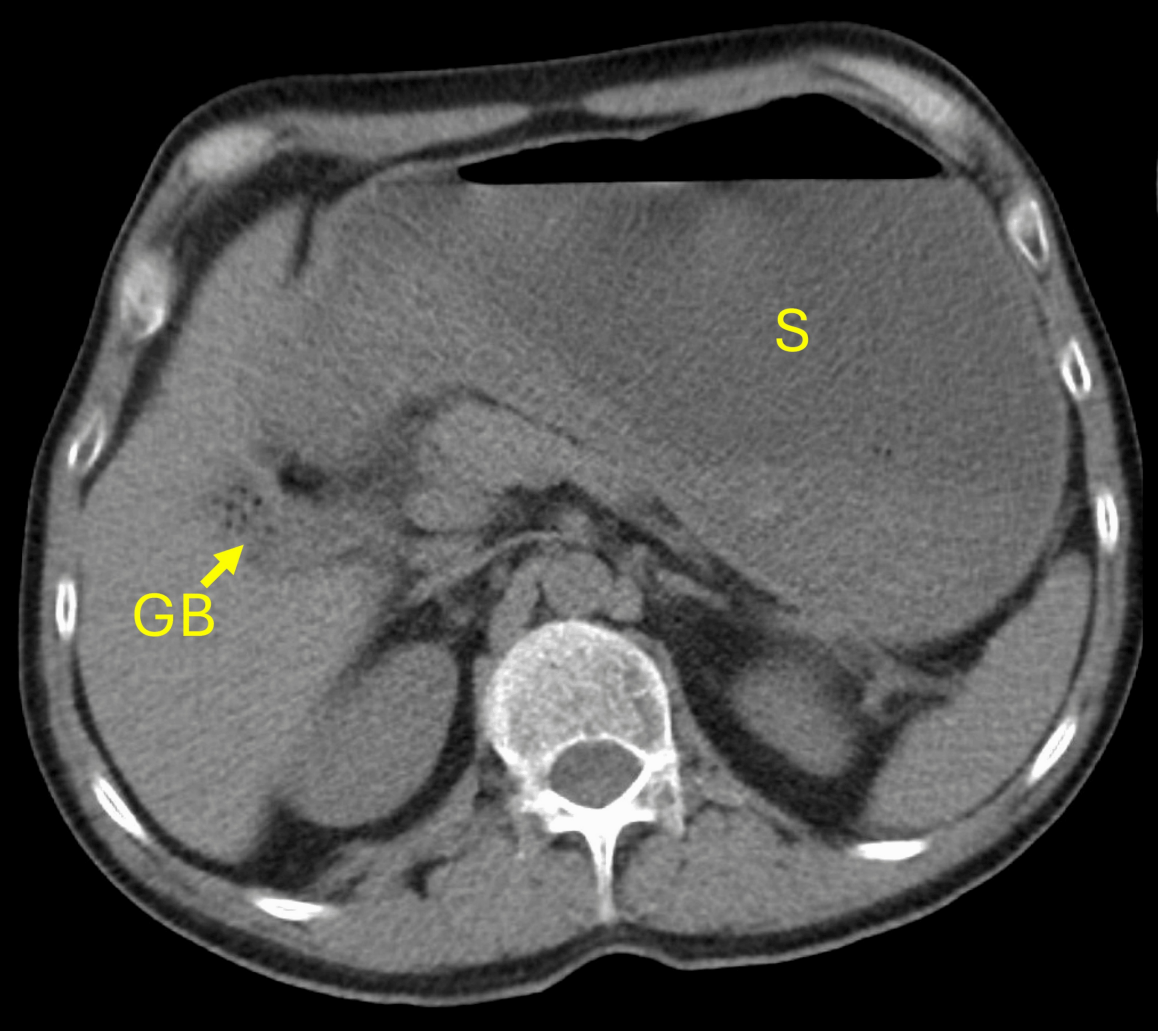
Imaging evaluation via CT scan of the abdomen Axial view of CT scan of the abdomen without oral contrast depicting the significantly distended stomach. S: stomach; GB: gallbladder; CT: computed tomography.
